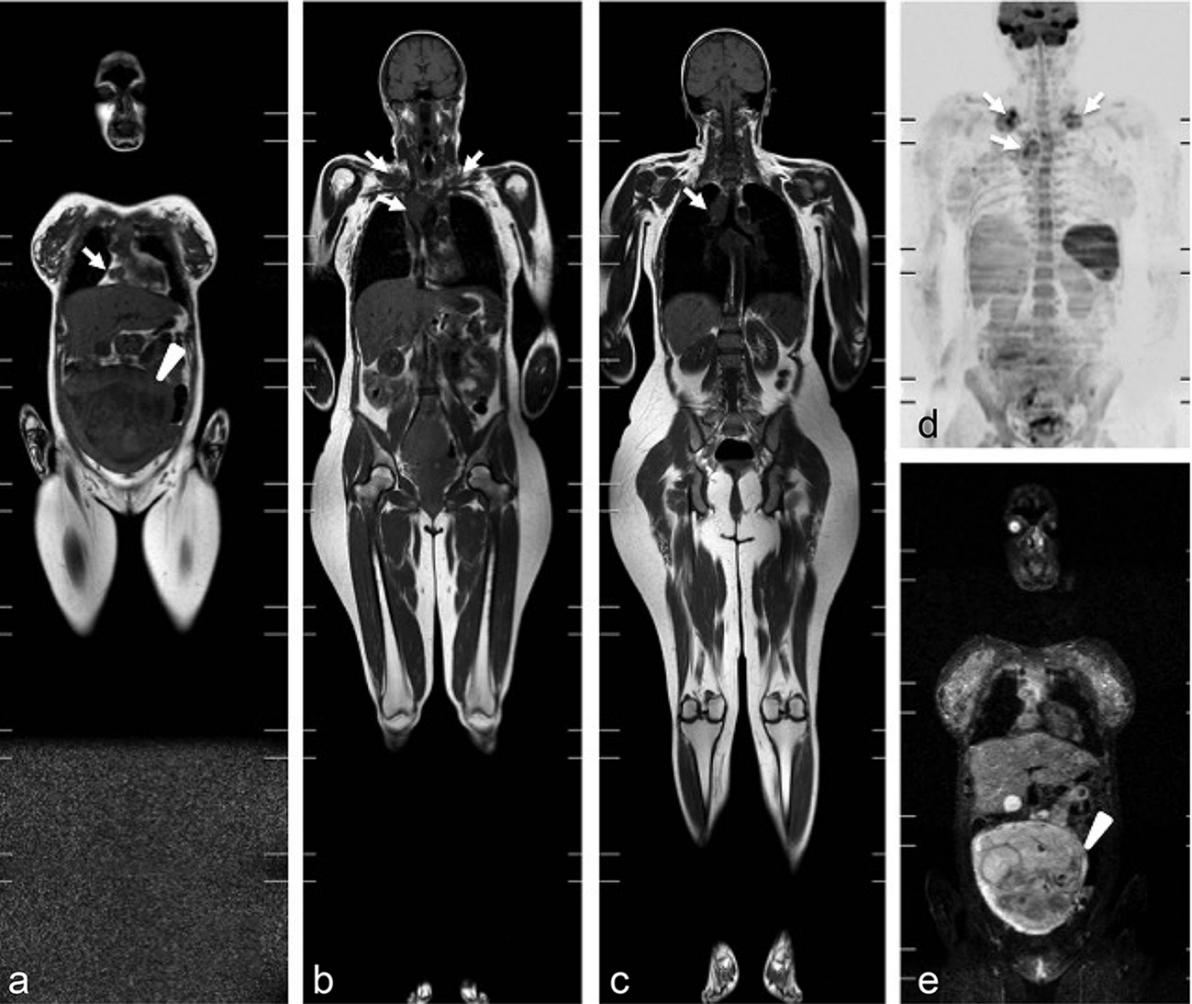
A 32-year-old pregnant female with Hodgkin’s lymphoma. Pregnancy week 23 (a, e; arrowheads). Coronal *T*_1_ weighted (a–c), coronal maximum intensity projection diffusion-weighted (d) and coronal short tau inversion-recovery (e) whole-body MRI showing bilateral supraclavicular, cardiophrenic and mediastinal lymph node involvement (a–d; arrows).
